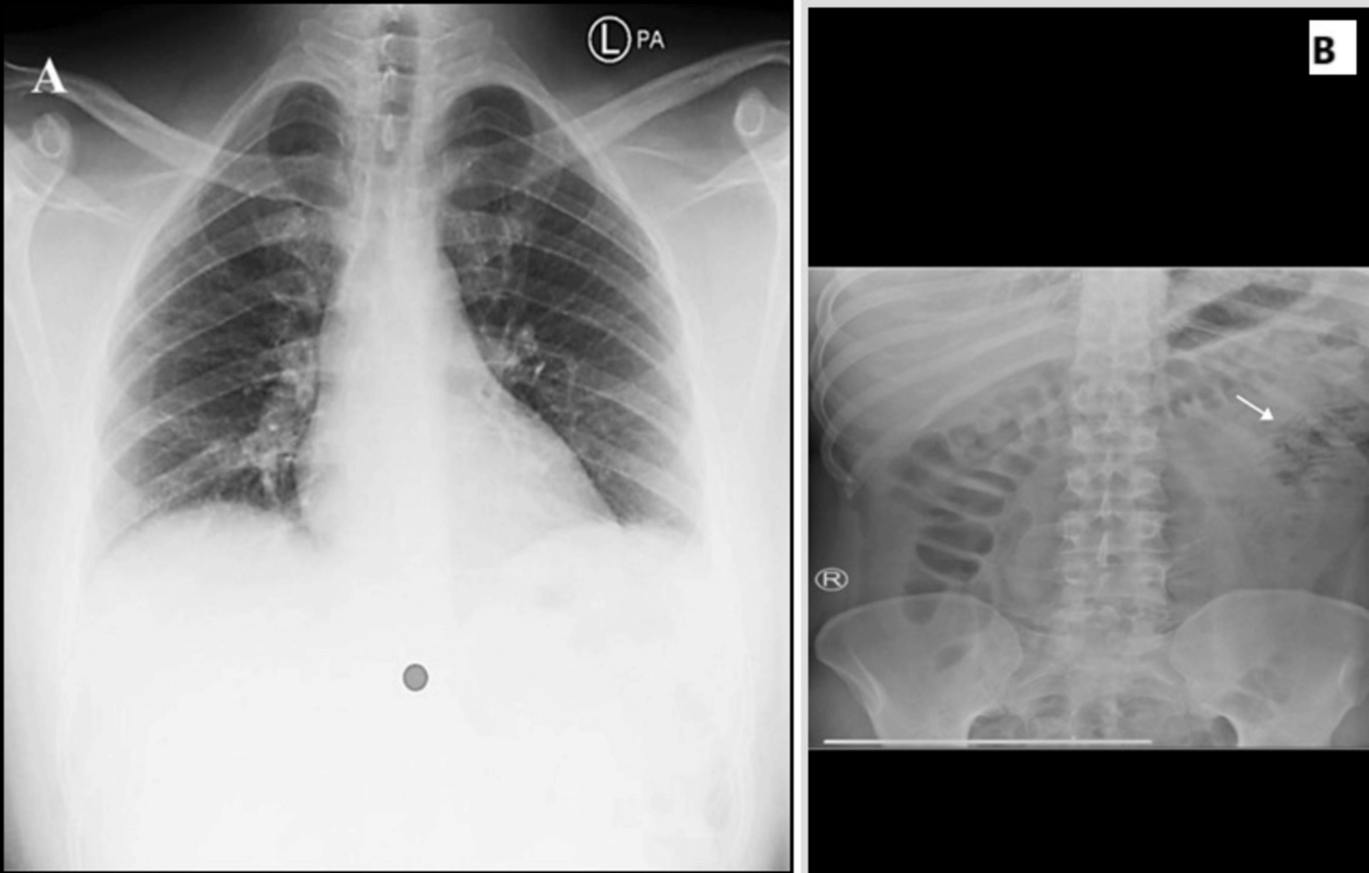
Chest postero-anterior (A) and abdominal (B) X-rays. An unremarkable chest and abdominal X-ray revealed a normal bowel loop diameter with Rigler’s sign.
